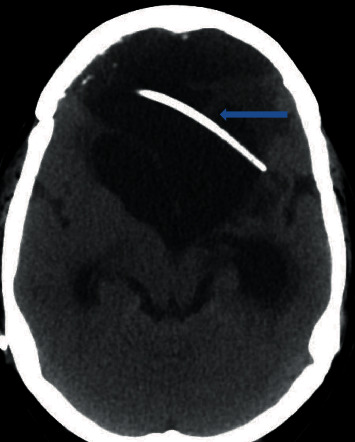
CT head showing preexisting VP shunt (blue arrow).
